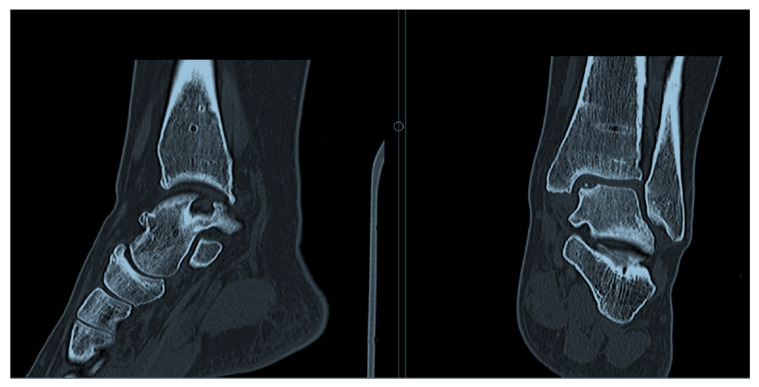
Representative CT scan sagittal and coronal view of a medial osteochondral lesion of the talus prior to treatment with AutoCart™.
